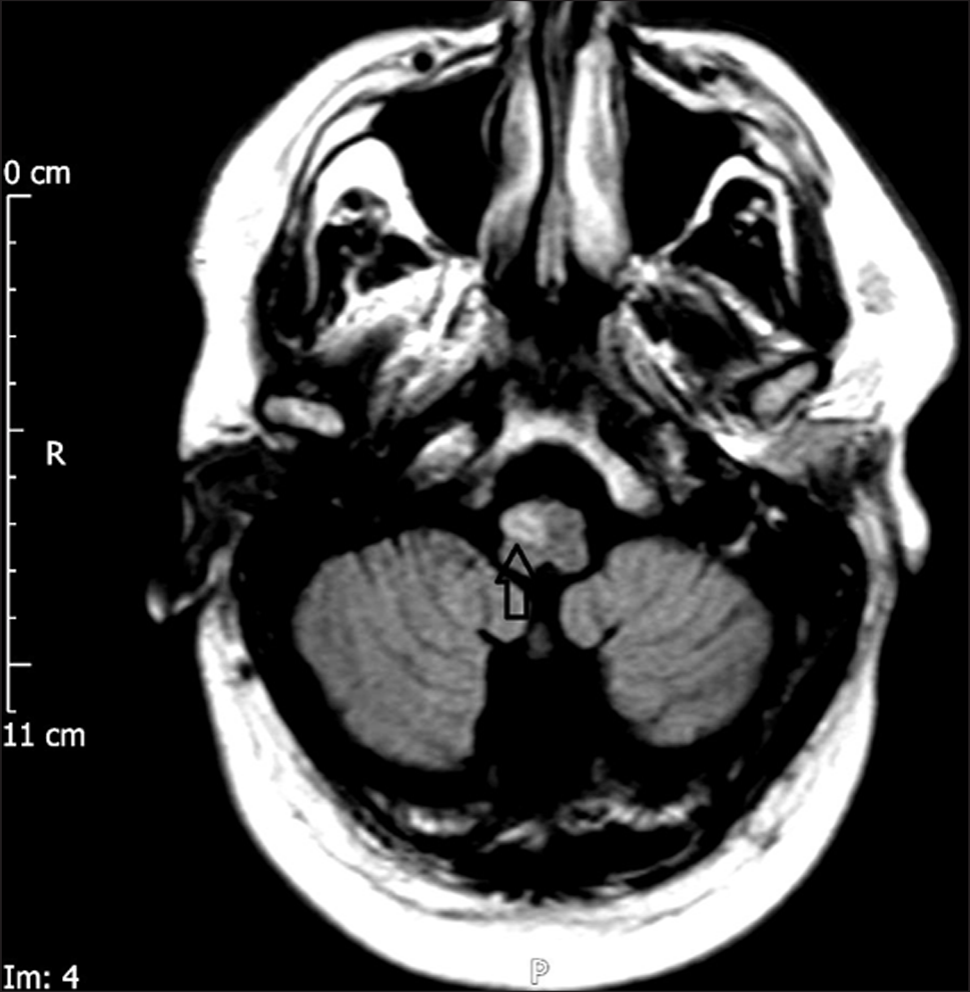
Axial T2 Weighted Fluid Attenuated Inversion Recovery (FLAIR) Magnetic Resonance Imaging (MRI) shows Increased Signal of the Inferior Olivary nucleus of the Right Hemi-medulla (black arrow) Suggestive of the Hypertrophic Olivary Degeneration.
